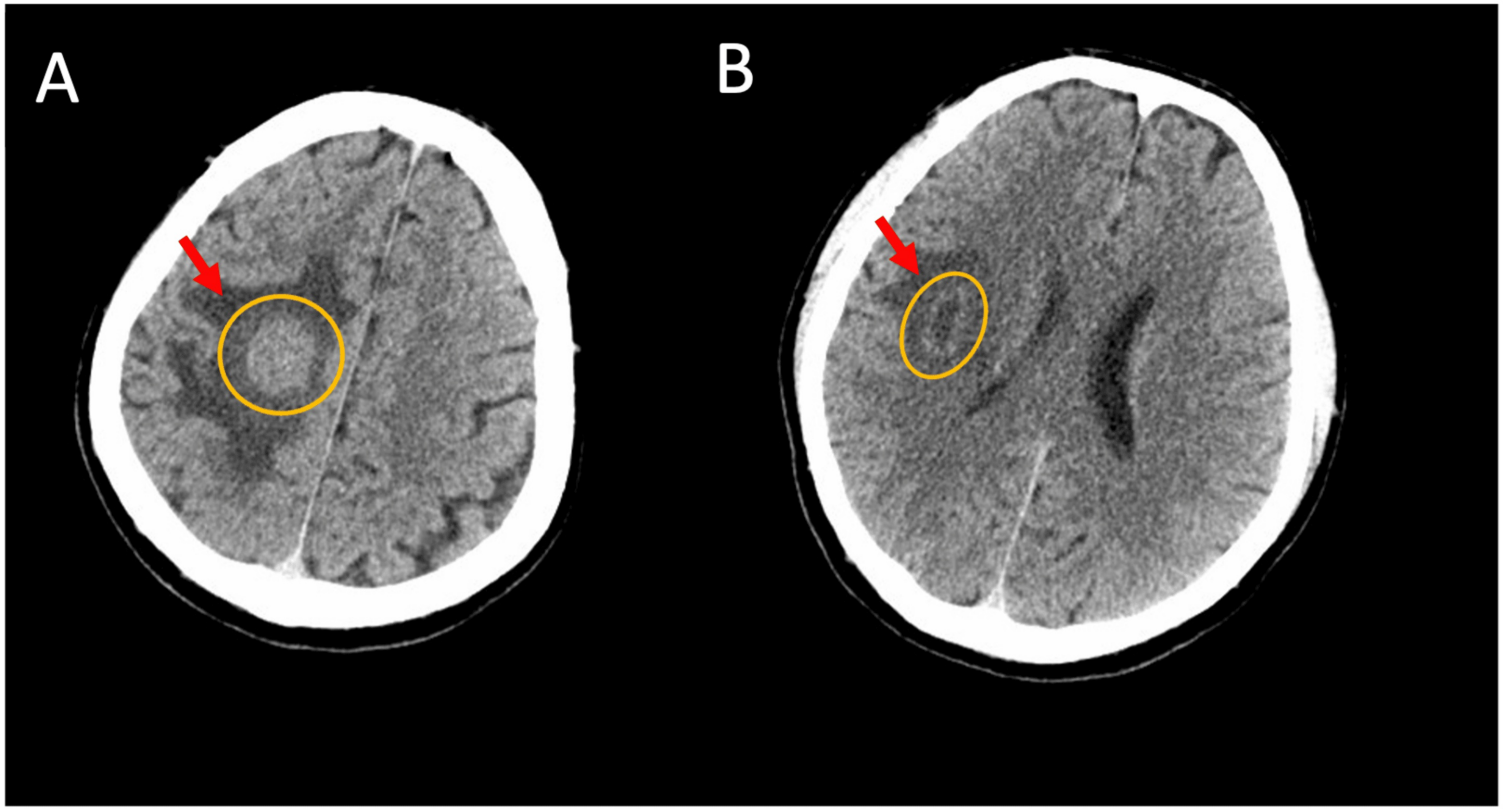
Axial CT scan (A) Right frontal lobe glioblastoma 3.5 cm × 3.1 cm (orange) surrounded by edema (red arrow); (B) 2.5 cm × 1.1 cm glioblastoma (orange) and edema (red arrow)
